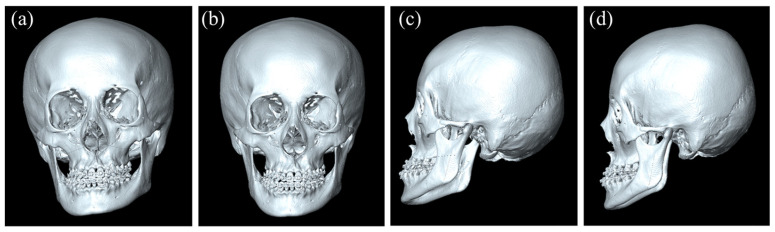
Three-dimensional skull mesh from CT scans before NHP reproduction (a) and after NHP reproduction (b) in the front view. Three-dimensional skull mesh from CT scans before NHP reproduction (c) and after NHP reproduction (d) in the side view.
